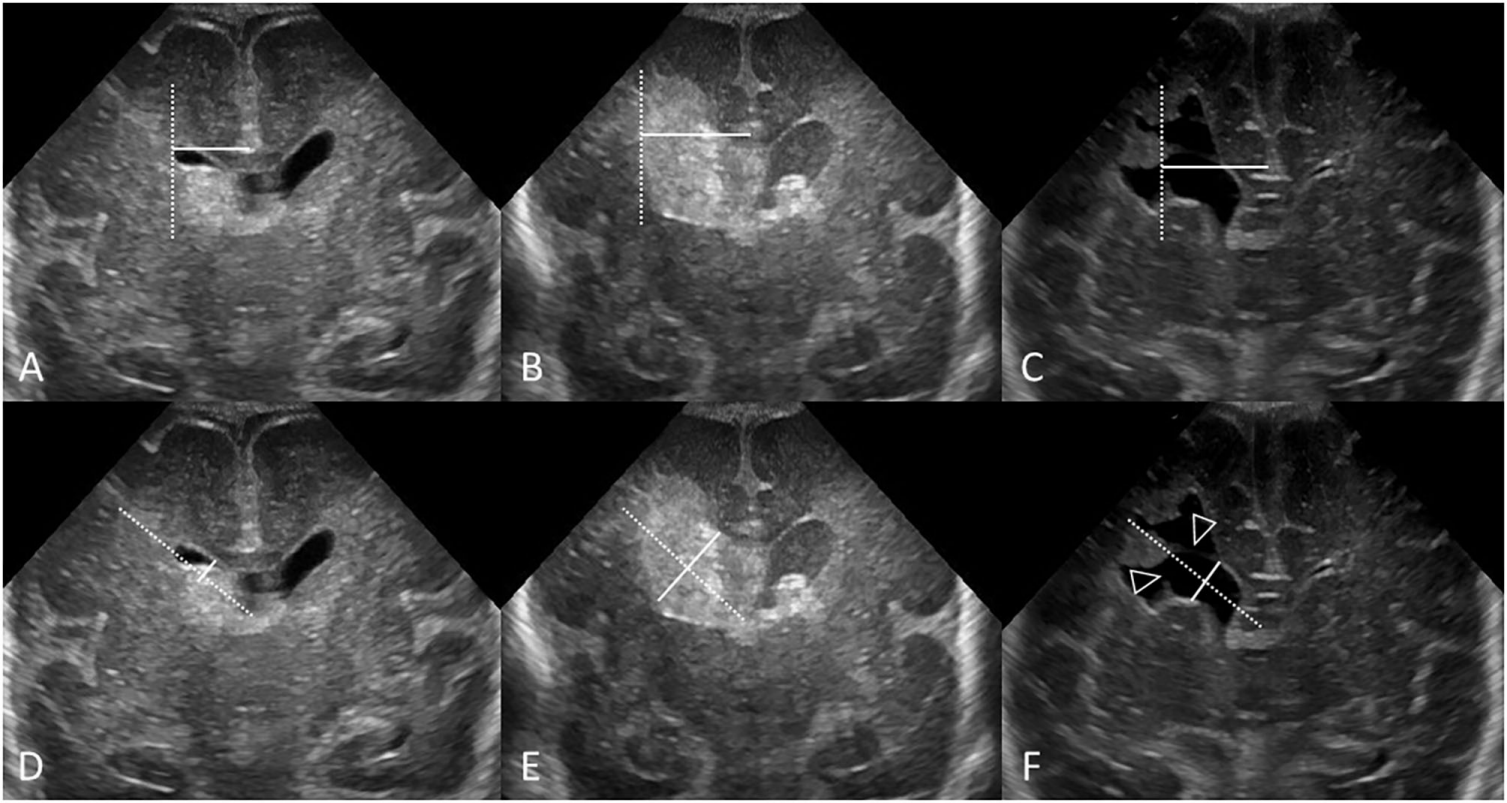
Measuring VI (A–C) and AHW (D–F) with obscured ventricular borders. Coronal ultrasound scans in 3 different preterm infants at the level of the frontal horns of the lateral ventricles. (A–C) VI measurements (solid line) and reference aid (dotted line). (D–F) Anterior horn width measurement (solid line) and reference aid (dotted line). It can occasionally be challenging to measure ventricular size when the ependymal margin of the ventricle is either obscured by echogenic clot (B,E) or interrupted by porencephalic cyst (C,F). (A,D) Grade I GMH-IVH. Small clot at the caudothalamic notch causes local extrinsic mass effect, “pushes” into, and partially effaces the frontal horn of the lateral ventricle. In cases like this, the AHW measurement should extend partially across the germinal matrix clot to reach the imaginary continuation of the lower ventricular wall as its reference point. (B,E) Grade III GMH-IVH with frontal PVHI. In this example, the correctly measured AHW will extend partially across the echogenic clot, but not include the portion that is believed intraparenchymal in location. (C,F) Porencephalic cyst. A ghost outline of the ventricle can occasionally still be seen (arrowheads) and aids definition of ventricular size. In this example, the correctly positioned reference aid across the long axis of the ventricle will assist measurement of the AHW (placed perpendicular to the reference aid).
